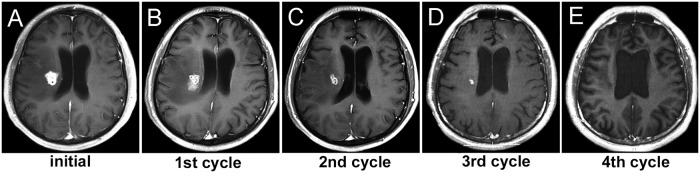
Dynamic cerebral MRI changes after praziquantel treatment. A 46-year-old male patient presented with left hemiplegia for three weeks and was diagnosed as cerebral sparganosis. He was treated with praziquantel (50mg/kg/day) in three divided doses for 10 days, and then the treatment cycle was repeated with monthly intervals until the active lesion completely disappeared on enhanced MRI. The initial enhanced MRI showed the aggregated ring-like lesions combined with severe edema at the right of periventricular (A). After the first treatment cycle, the lesion reduced a little in size (B). After the second treatment cycle, the lesion was obviously smaller (C). After the third treatment cycle, the lesion showed a resolution of swelling (D). After the fourth treatment cycle, the lesion had completely disappeared (E).
